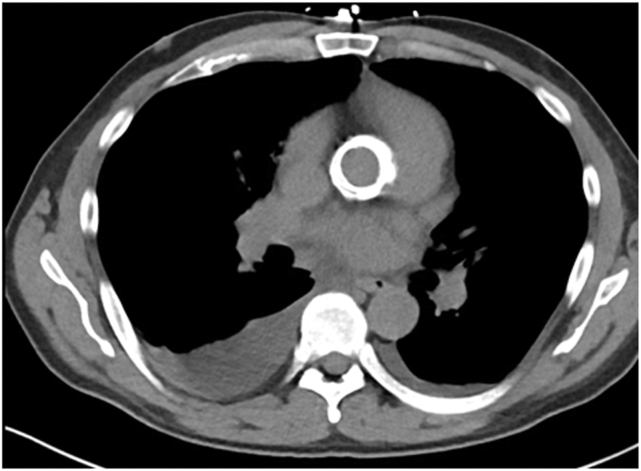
Preoperative CT scan. Axial image showing circumferential aortic root calcification. *CT*, Computed tomography.
